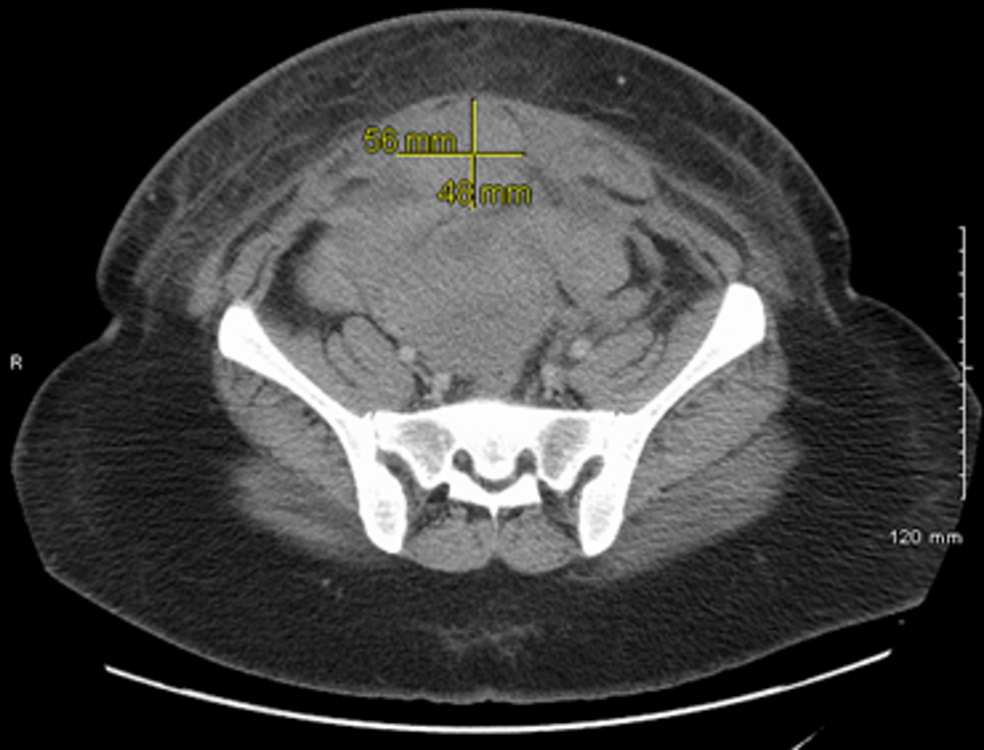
Computed tomography axial view showing the hematoma anterior to the surgical line of the uterus (5.6 cm x 4.8 cm)
